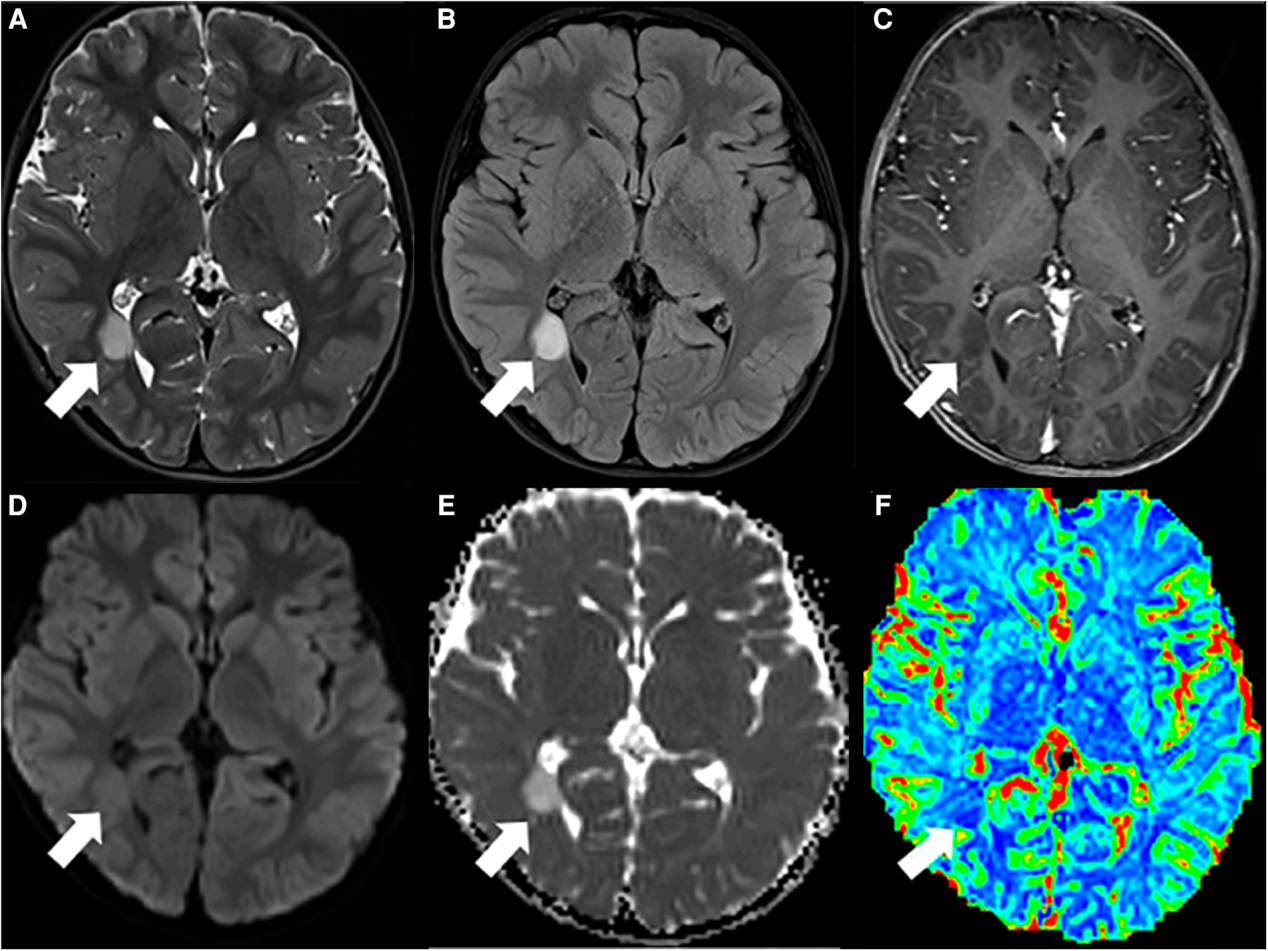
Pediatric-type diffuse low-grade glioma. (A) T_2_WI and (B) FLAIR hyperintense lesion in the right temporo-occipital (arrow). (C) There is no enhancement on the T_1_WI postcontrast image (arrow). (D) Facilitated diffusion seen on diffusion-weighted imaging (DWI) (arrow) and (E) ADC map (arrow). (F) Hypoperfusion is seen on the rCBV map (arrow). This figure was originally posted by Kalelioglu et al. in the *Neuroradiology Journal* in September 2022 (9).
